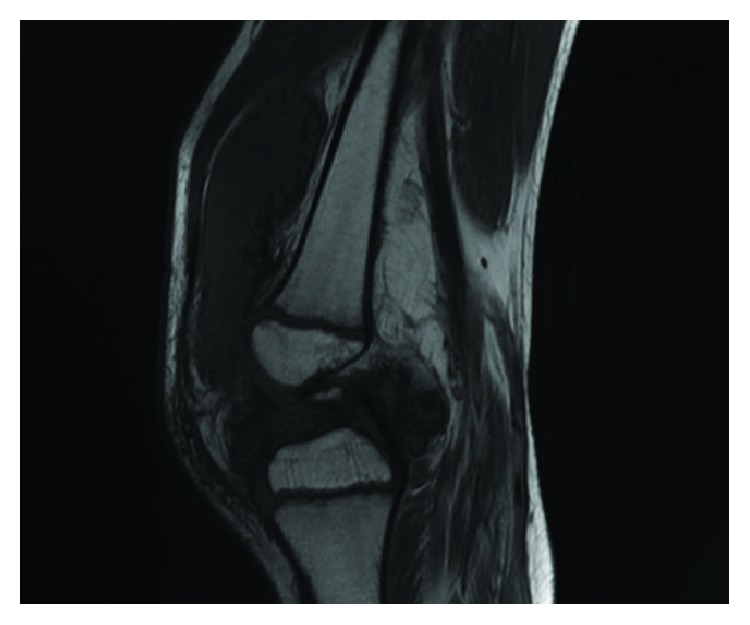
MRI, T1 sequence of the left knee: visible villonodular thickening of the synovial membrane with hemosiderin deposition.
